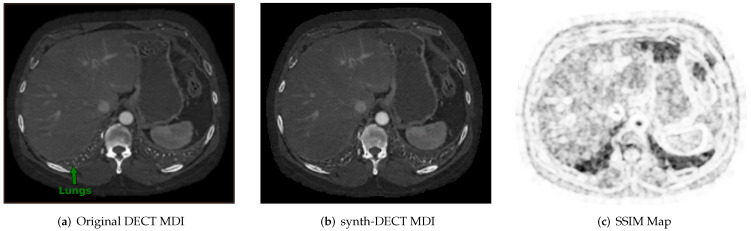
Example cross sectional axial slices from the test dataset used for Pix2Pix. (a) The original dual energy CT material density iodine (DECT MDI). (b) The synth-DECT MDI for the slice shown in (a). The global structural similarity index (SSIM) for the scan from which the slices were taken was computed to be 0.92. (c) This figure displays the local SSIM scores for each pixel of the slices in (a,b) as an image: The dark areas depict small values of the SSIM, which indicates a large difference between the original and synthetic image. The bright regions show large values of the SSIM or areas that were the most similar between the original and synthetic.
